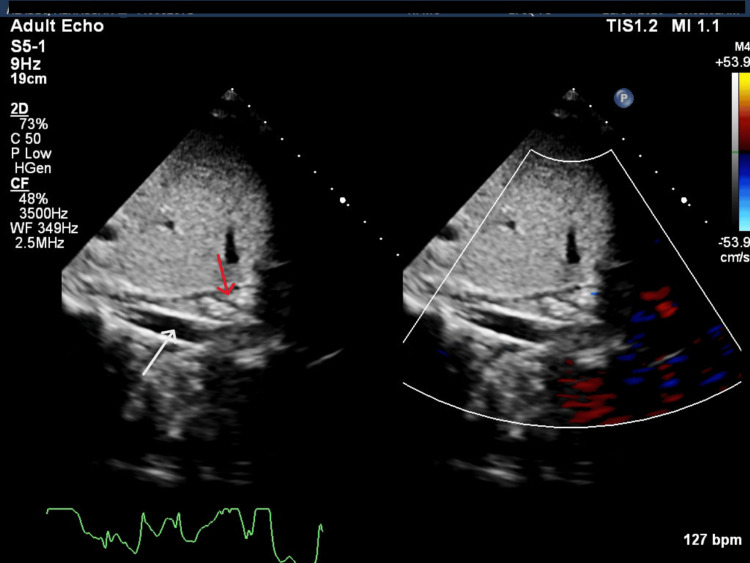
Subcostal echocardiographic view demonstrating the inferior vena cava (IVC) Subcostal echocardiographic view showing major abdominal vessels. The white arrow indicates the aorta, while the red arrow indicates the obliterated IVC.
